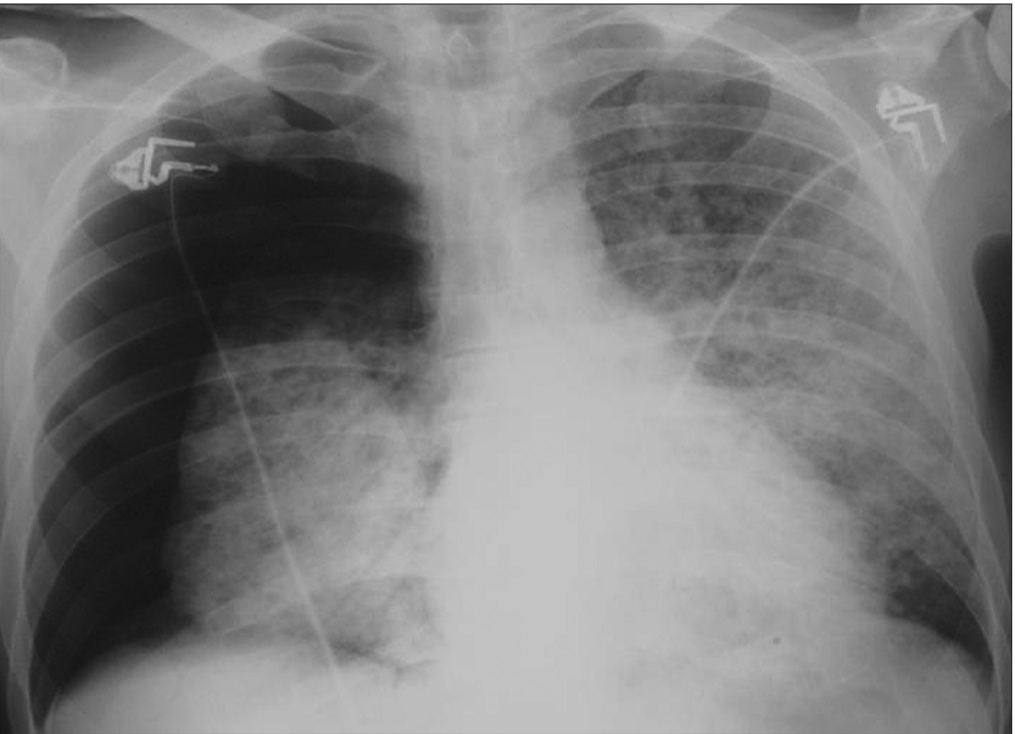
Pneumocystis carinii* pneumonia. Chest X-ray showing an approximately 50% right-sided spontaneous pneumothorax
